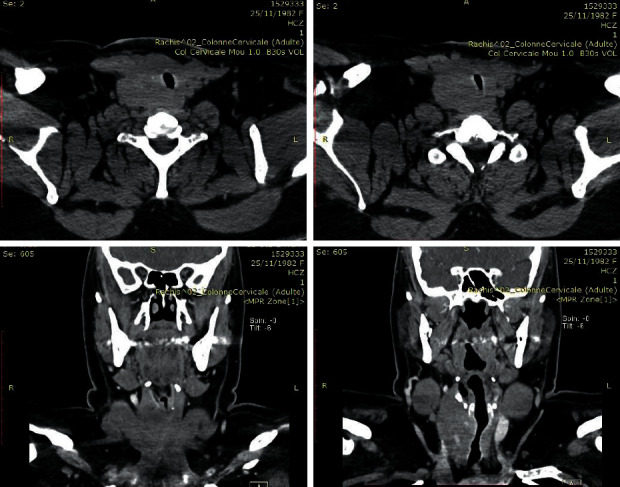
CT image in transverse and coronal sections showing a large goiter with tracheal deviation and stenosis.
